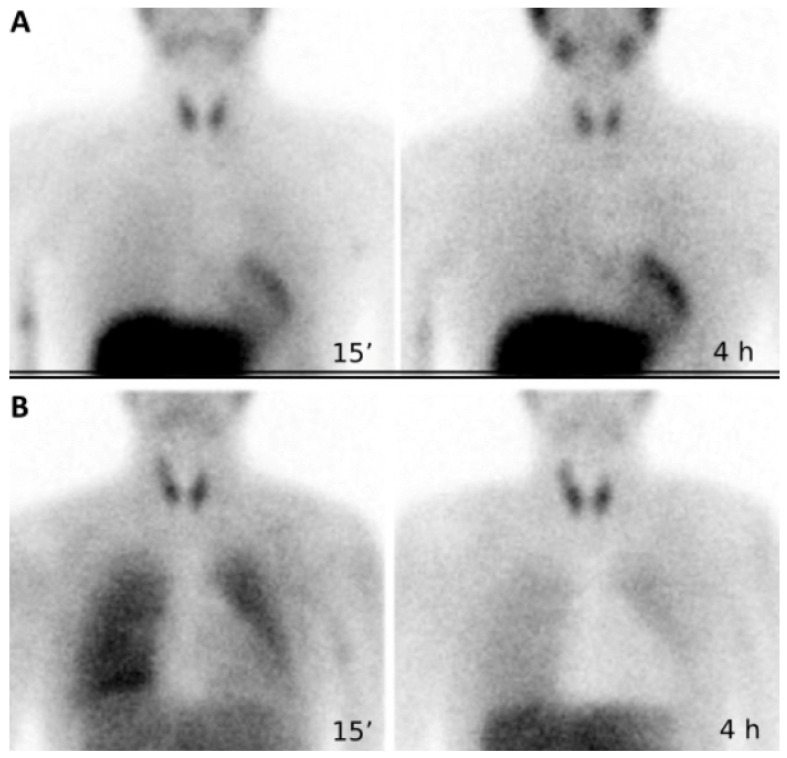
^123^I-mIBG scintigraphy is a useful method to assess cardiac reinnervation in pediatric patients undergoing heart transplantation, improving their clinical management. (A): Early and delayed images in the upper panel showed a significant myocardial ^123^I-mIBG uptake in a patient without chest pain who underwent heart transplantation for dilated cardiomyopathy. (B): No significant myocardial ^123^I-mIBG uptake is evident in images displayed in the lower panel. The patient underwent heart transplantation (9 years before) for univentricular heart, asymptomatic for chest pain, had a sudden cardiac death 8 months after ^123^I-mIBG scintigraphy.
